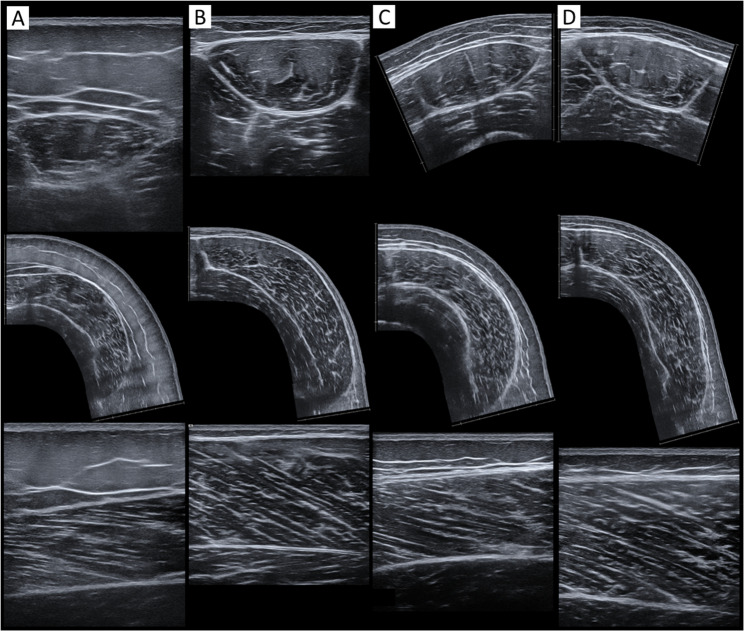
Panoramic (top and middle row) and static (bottom row) Ultrasonography images of the m. rectus femoris (top row) and m. Vastus lateralis (middle row) anatomical cross-sectional area as well as m. vastus lateralis architecture (bottom row) of cerebral palsy untrained (A), cerebral palsy trained (B), healthy untrained (C) and healthy trained (D) females. The images were taken at 50% of femur length in the dominant (left) leg of the participants
